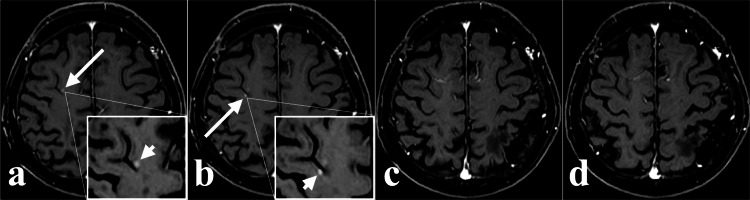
Rapid response of the right frontal lobe lesions on axial enhanced MRI. (a, b) At 63 months postoperatively, follow-up MRI revealed two new intraparenchymal lesions across the precentral sulcus: (a) a 3-mm lesion in the middle frontal gyrus (arrow) and (b) a 2-mm lesion in the precentral gyrus (arrow). (c, d) At 65 months (2 months after low-dose SRT), follow-up MRI shows complete resolution of both lesions. MRI; magnetic resonance imaging, SRT; stereotactic radiotherapy
